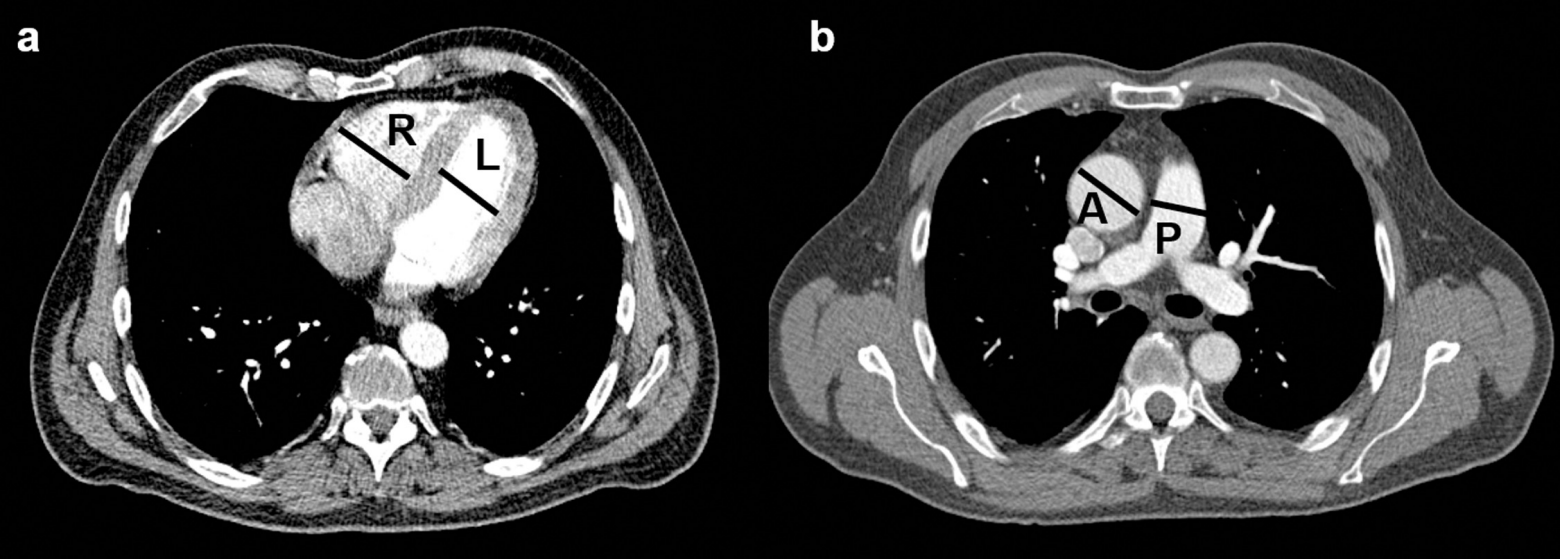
CT measurements. a) Right/left ventricular ratio was calculated by dividing the maximal distance between the ventricular endocardium and the interventricular septum, perpendicular to the long axis of the heart measured on standard axial views. The maximum dimensions for both ventricles where used for measurements. b) The pulmonary artery and ascending aorta were assessed on a transverse image at the level of the pulmonary artery bifurcation. Vessel diameters were obtained by measuring the widest diameter vertical to the long axis of the main pulmonary artery.
